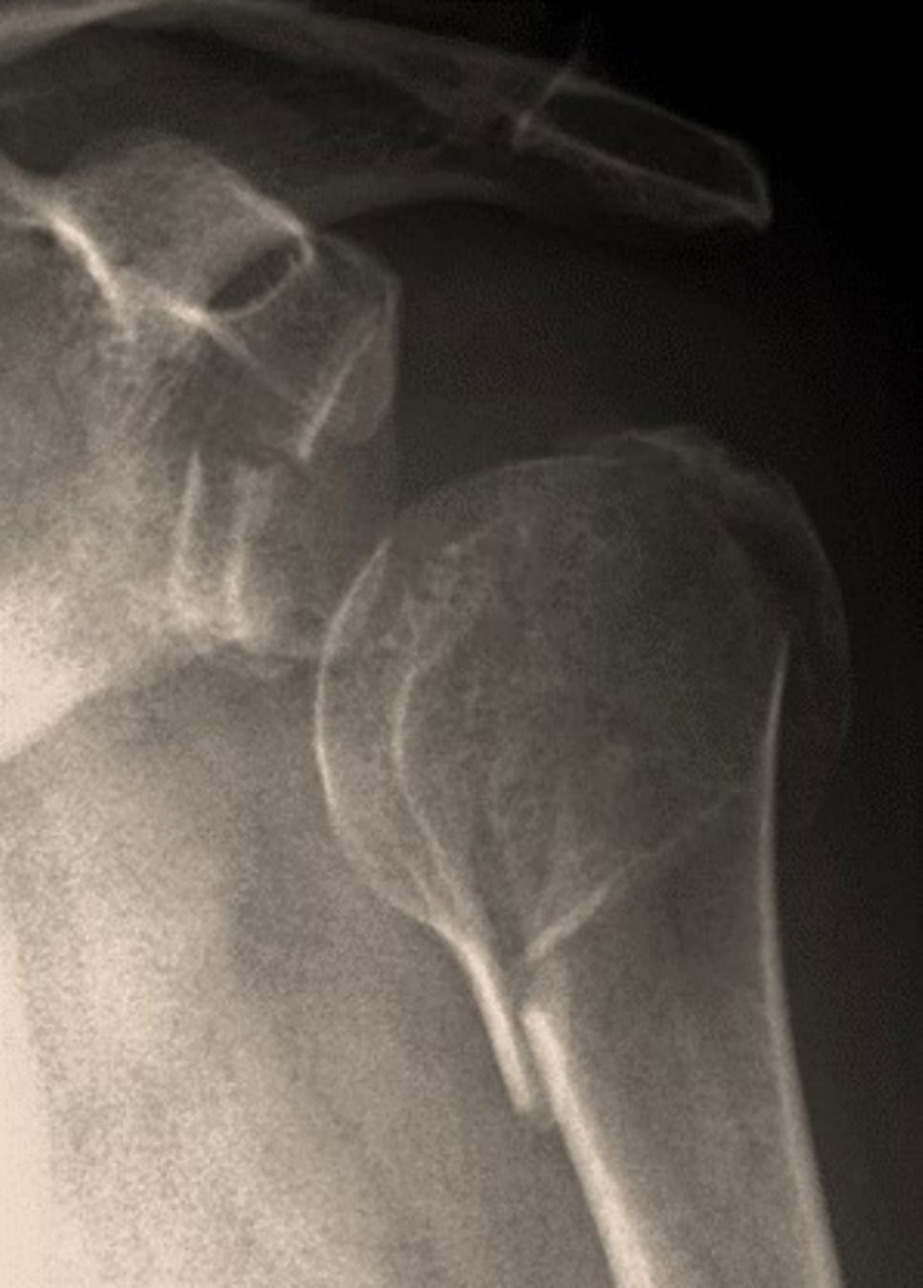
Glenohumeral combination fracture (proximal humerus and anterior glenoid rim fracture, a.p.-radiograph)
